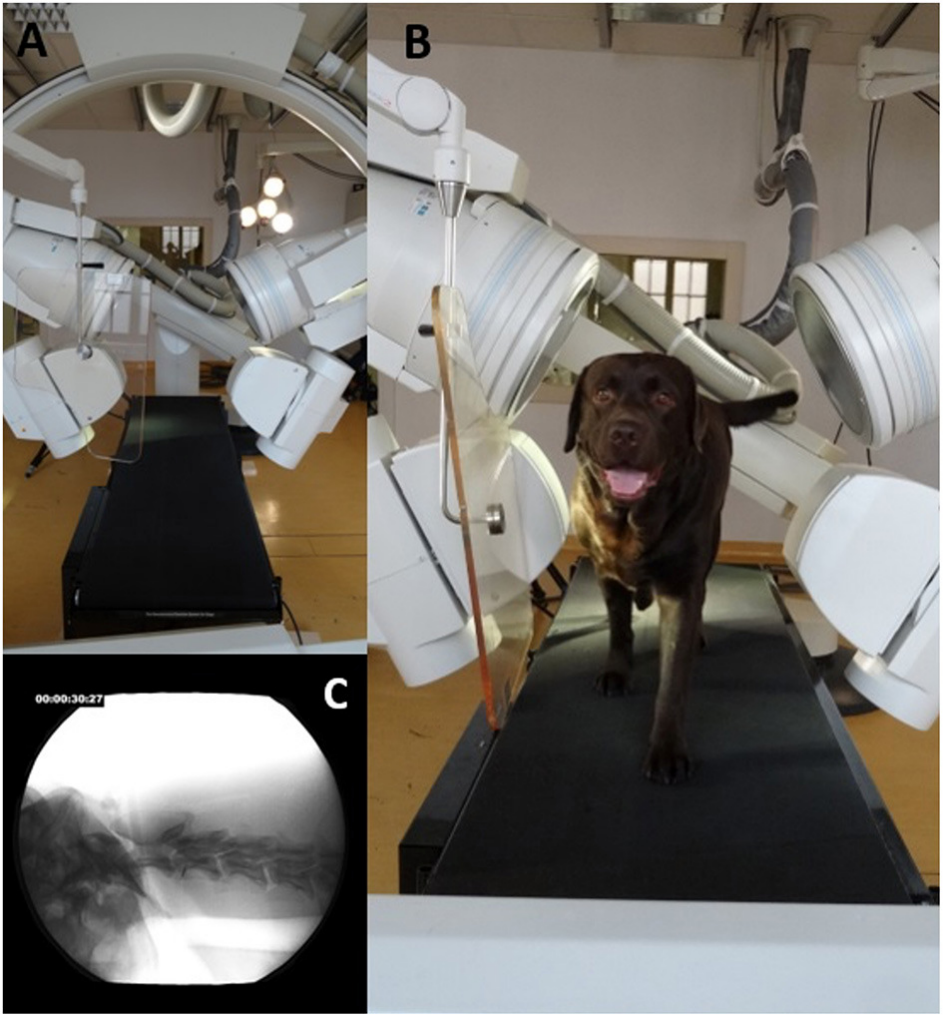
Fluoroscopy 63° experimental setup. (A): 63° fluoroscopy setting to record the oblique-laterolateral beam path. The treadmill is located in the center. (B): Labrador retriever walking on the treadmill located in the beam path. (C): X-ray video view of the oblique-laterolateral beam path.
